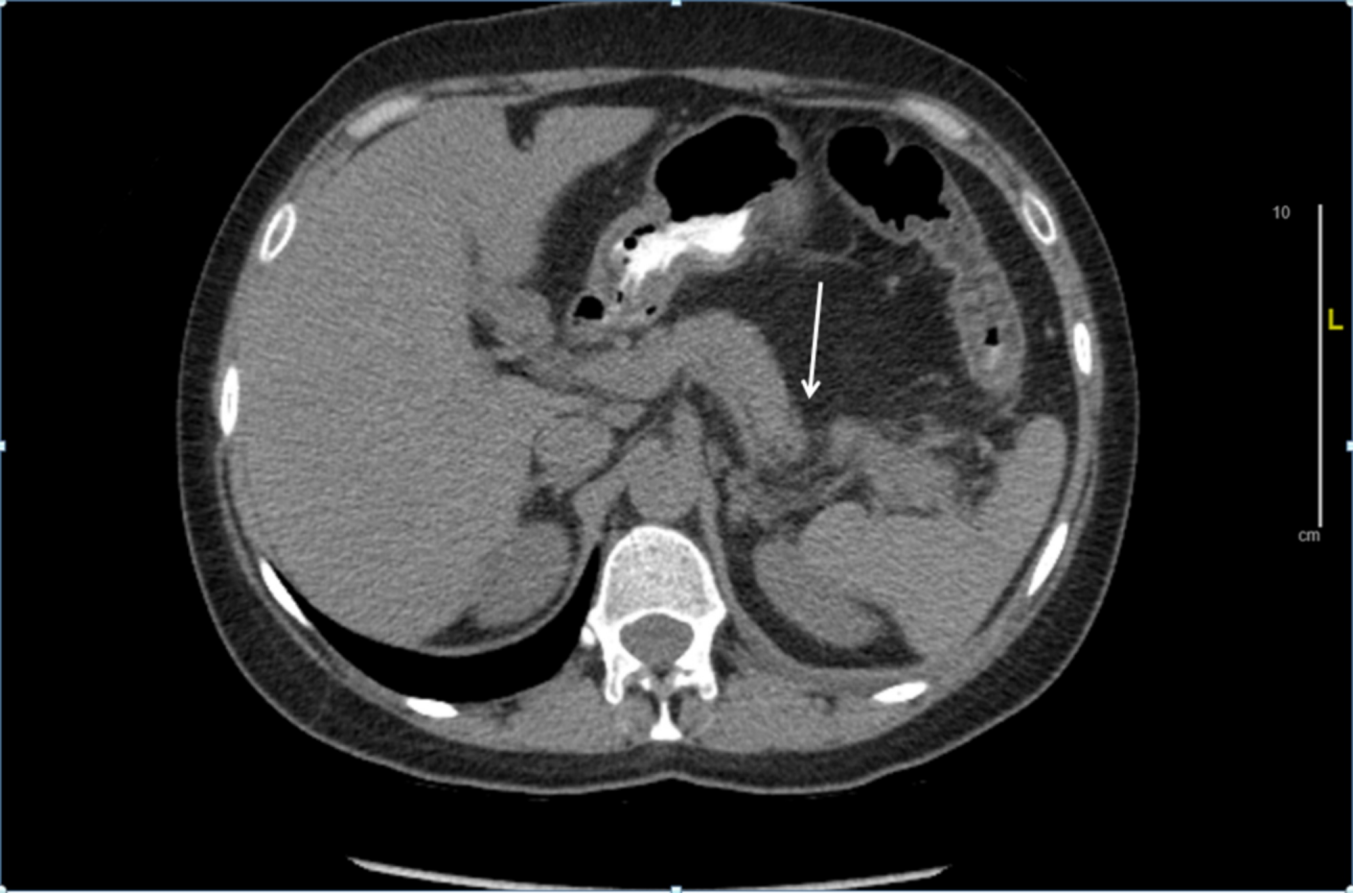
CT scan of the abdomen showing infiltration of mesenteric fat around the tail of the pancreas suggestive of acute pancreatitis
